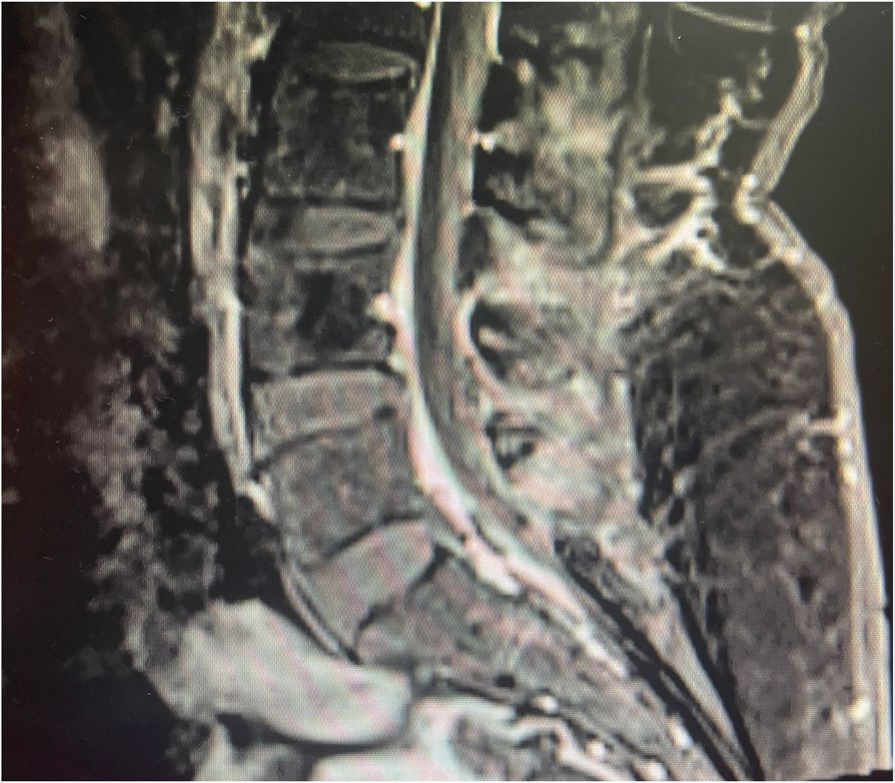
Sagittal image of the MRI spine revealing contrast enhancement of the cauda equina and anterior dura
